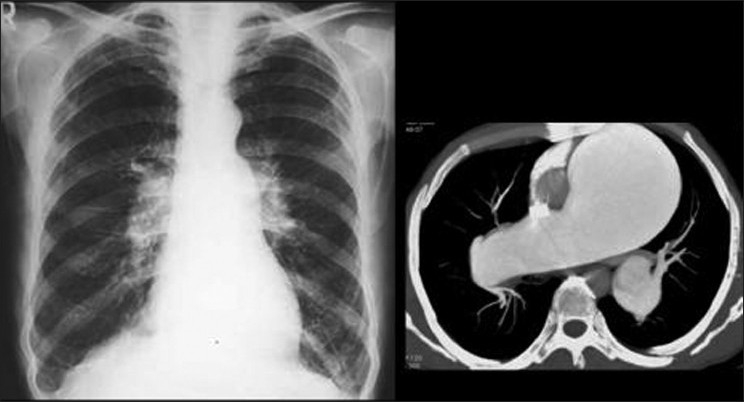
A CXR of a 41-year-old man with cirrhosis and PPH show enlarged hila and peripheral pruning of the vessels secondary to PH. An axial contrast enhanced CT through the pulmonary arteries of another patient with PPH showing aneurismal dilatation of the main pulmonary artery and the right pulmonary artery with peripheral pruning due to PH
